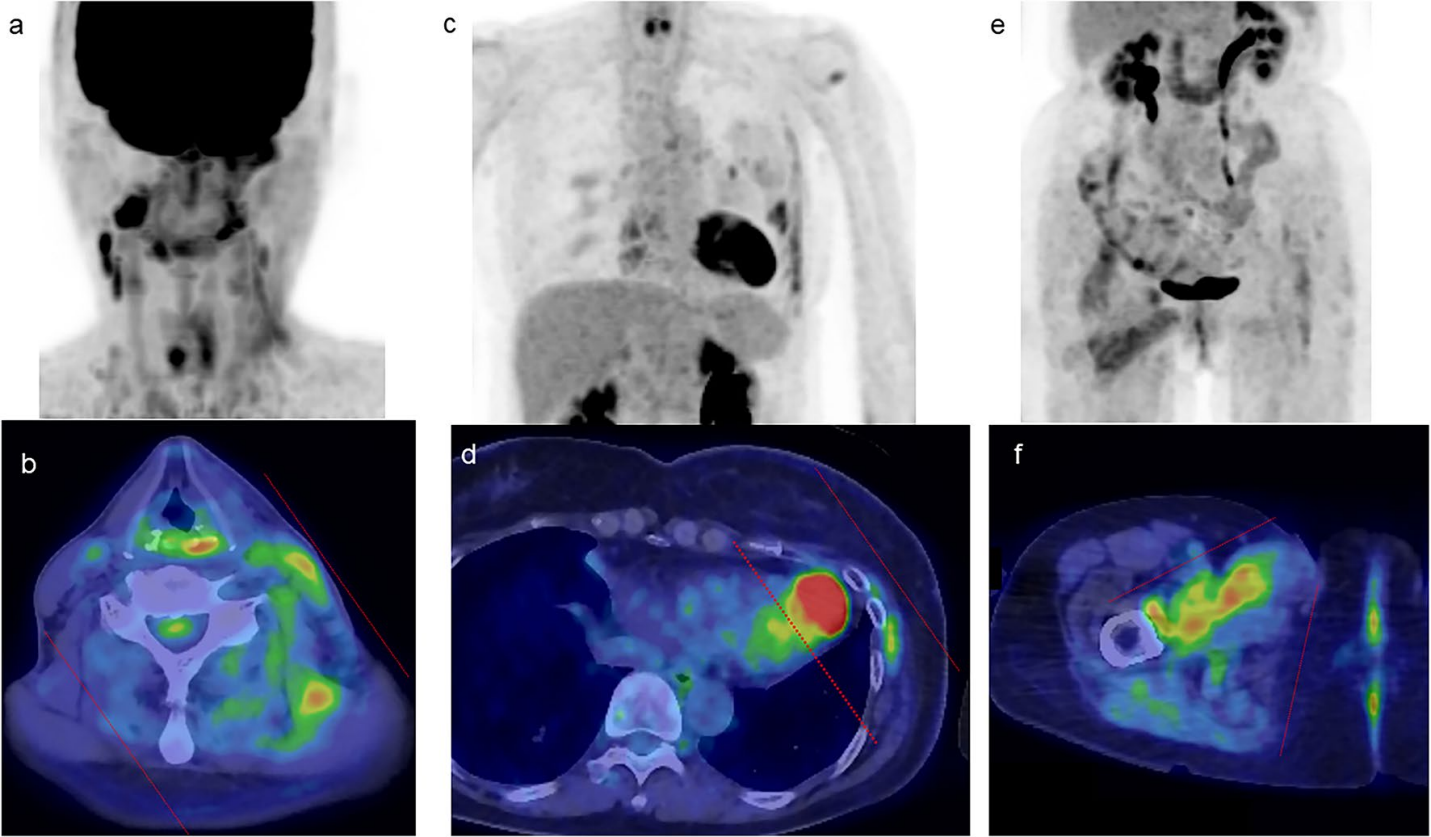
a, c, and e FDG-PET MIP images. b, d, and f Axial FDG-PET/CT images. The area of FDG uptake matches the field of radiation therapy (marked by dotted lines)
